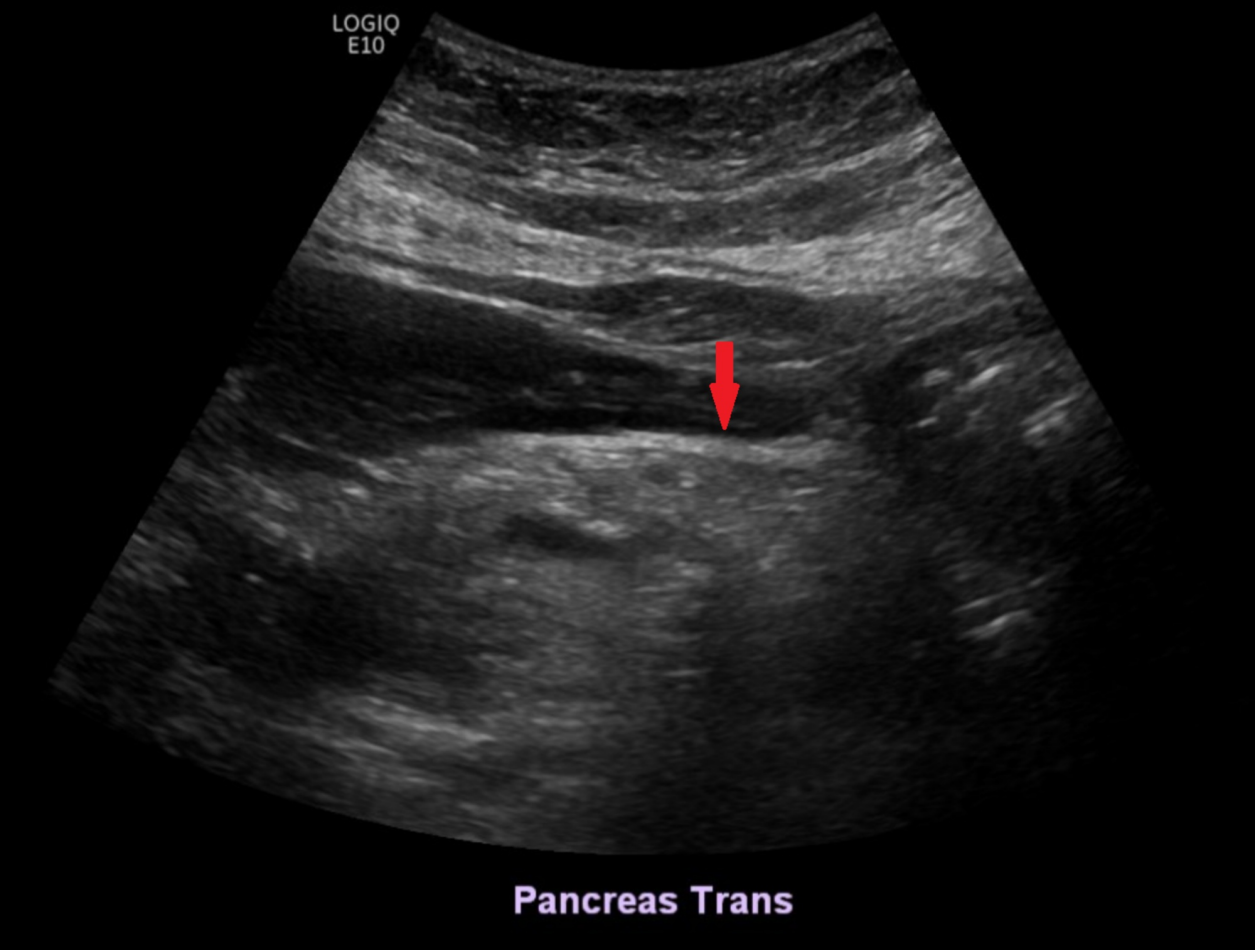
Ultrasound of the abdomen showing normal heterogeneous pancreas with hypo and hyperechogenicity of the parenchyma of the normal-sized pancreatic head and body, negative for parenchymal lesions or pancreatitis. The pancreatic tail was sub-optimally assessed due to overlying bowel gas.
